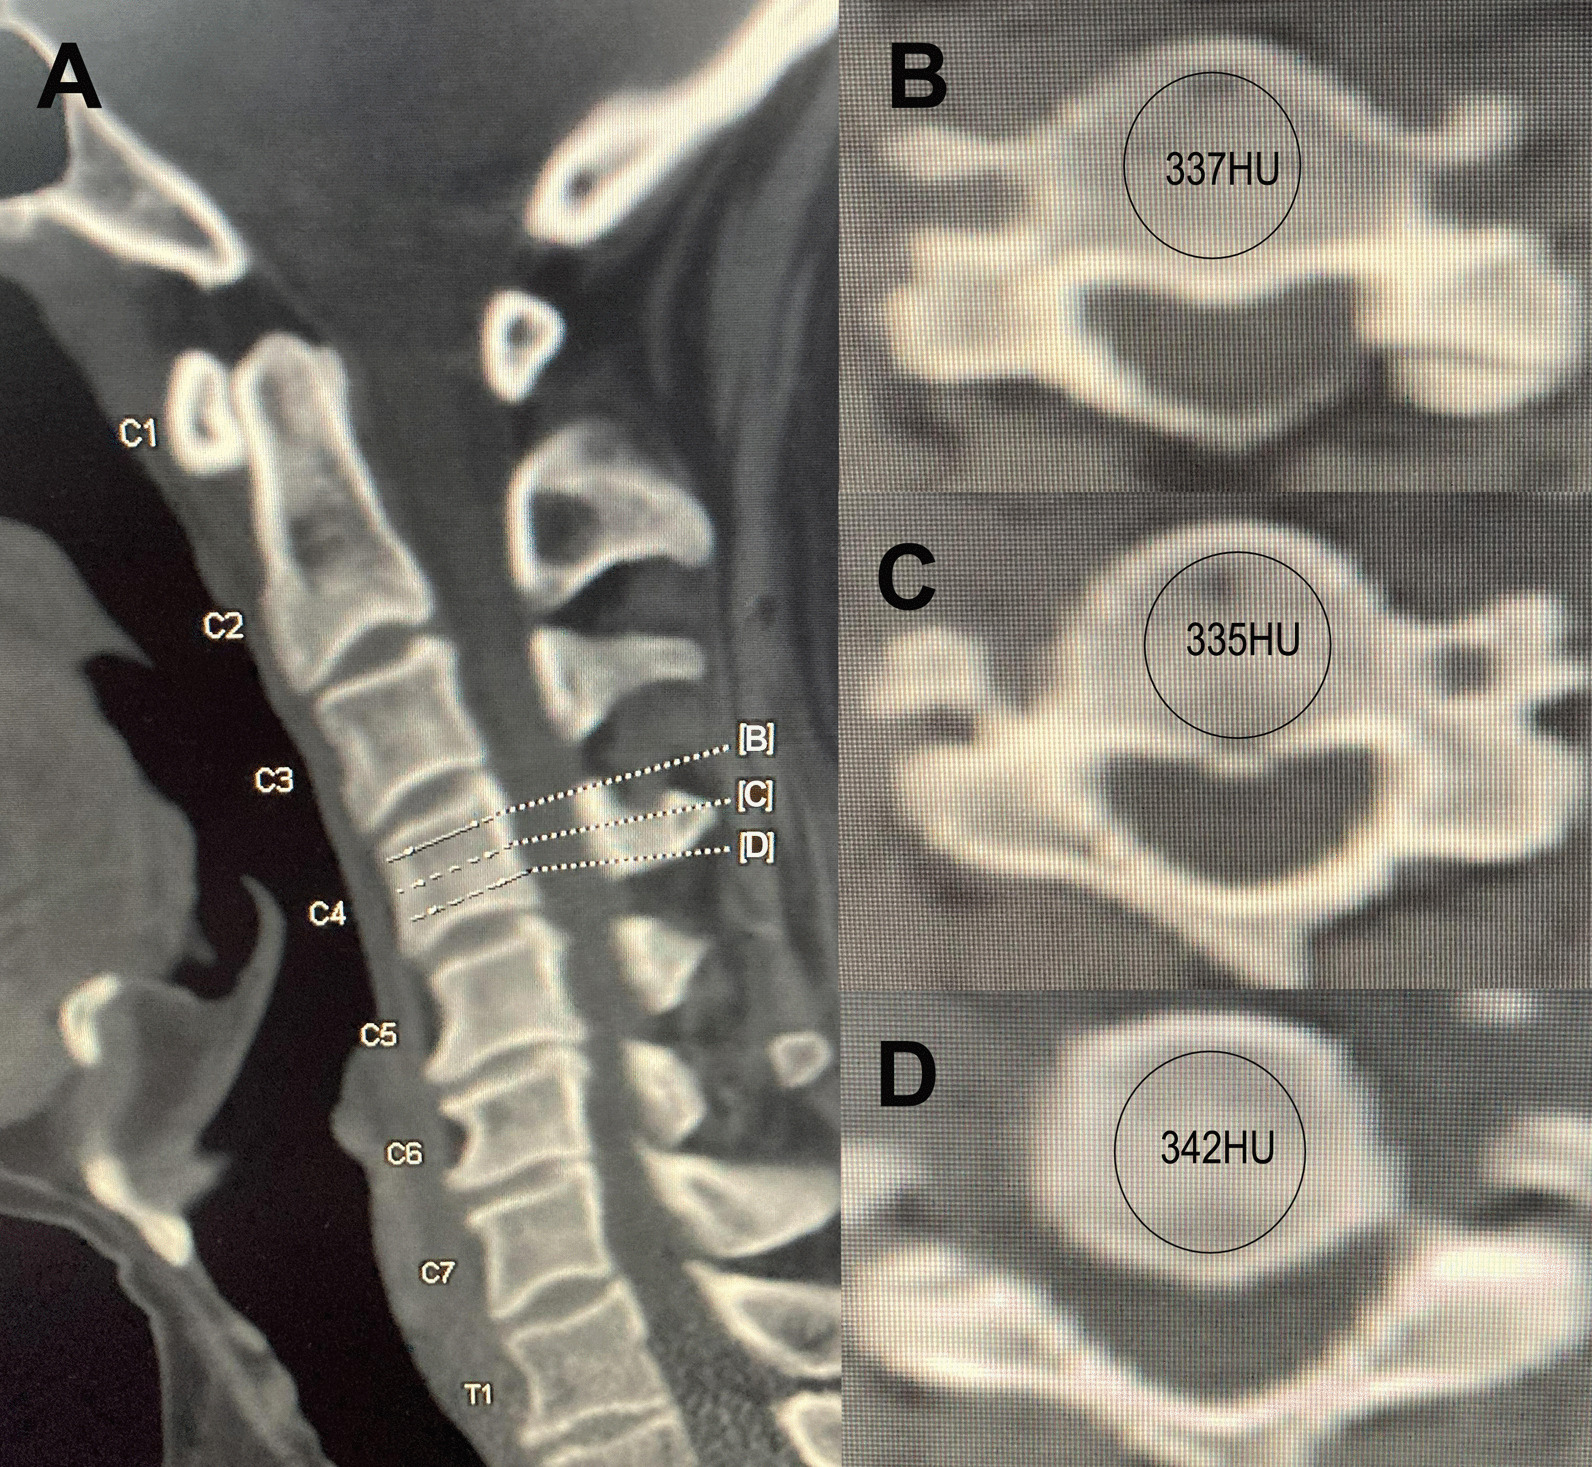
Midsagittal (A) and axial CT images demonstrating the measurement of vertebral HU value on the axial plane inferior only to the superior endplate (B), middle of the vertebral (C) and axial plane superior only to the inferior endplate (D) (the first letter C stands for cervical vertebra, which consists of seven segments from top to bottom, denoted by C1-C7, the same as T1)
